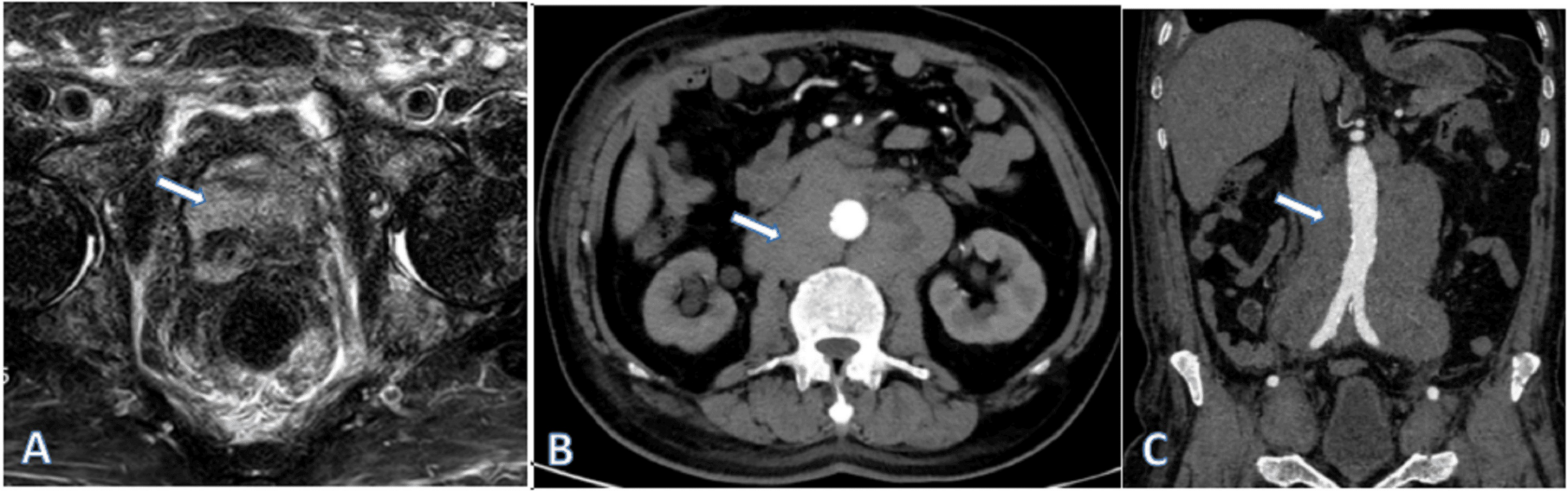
Prostate MRI and abdominal CT enhanced scan images. (A) MRI showing abnormal signals in the prostate. (B and C) Enhanced CT showing multiple enlarged lymph nodes around the abdominal aorta with uneven enhancement MRI: magnetic resonance imaging, CT: computed tomography
